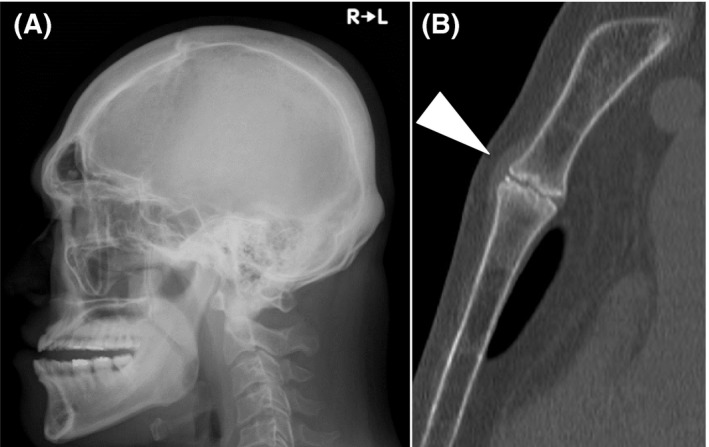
(A) Head X‐ray showed enlarged sinuses and an extended lower jaw. (B) Chest CT (sagittal section) showed irregularities of the joint spaces (white arrowhead).
